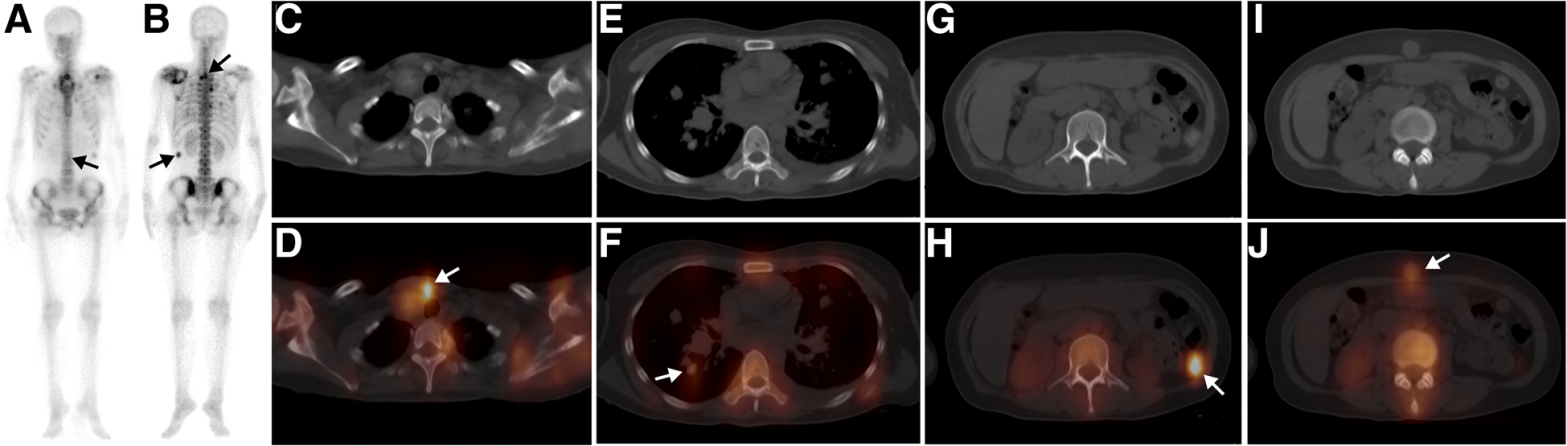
A 59-year-old man with known scapular osteosarcoma was referred for metastatic work-up. The whole-body scan (a, anterior; b, posterior) showed multiple foci of abnormally increased ^99m^Tc-MDP uptake in the left shoulder, supraclavicular region, chest and abdomen (black arrow). Axial CT (c, e, g, i), and SPECT/ CT images (d, f, h, j) showed that the elevated ^99m^Tc-MDP activity was located in the left shoulder, supraclavicular lymph nodes, pleura, lung, posterior peritoneum and periumbilical subcutaneous tissue (the lesion was missed on WBS due to overlap with the lumbar vertebra) (white arrow)
